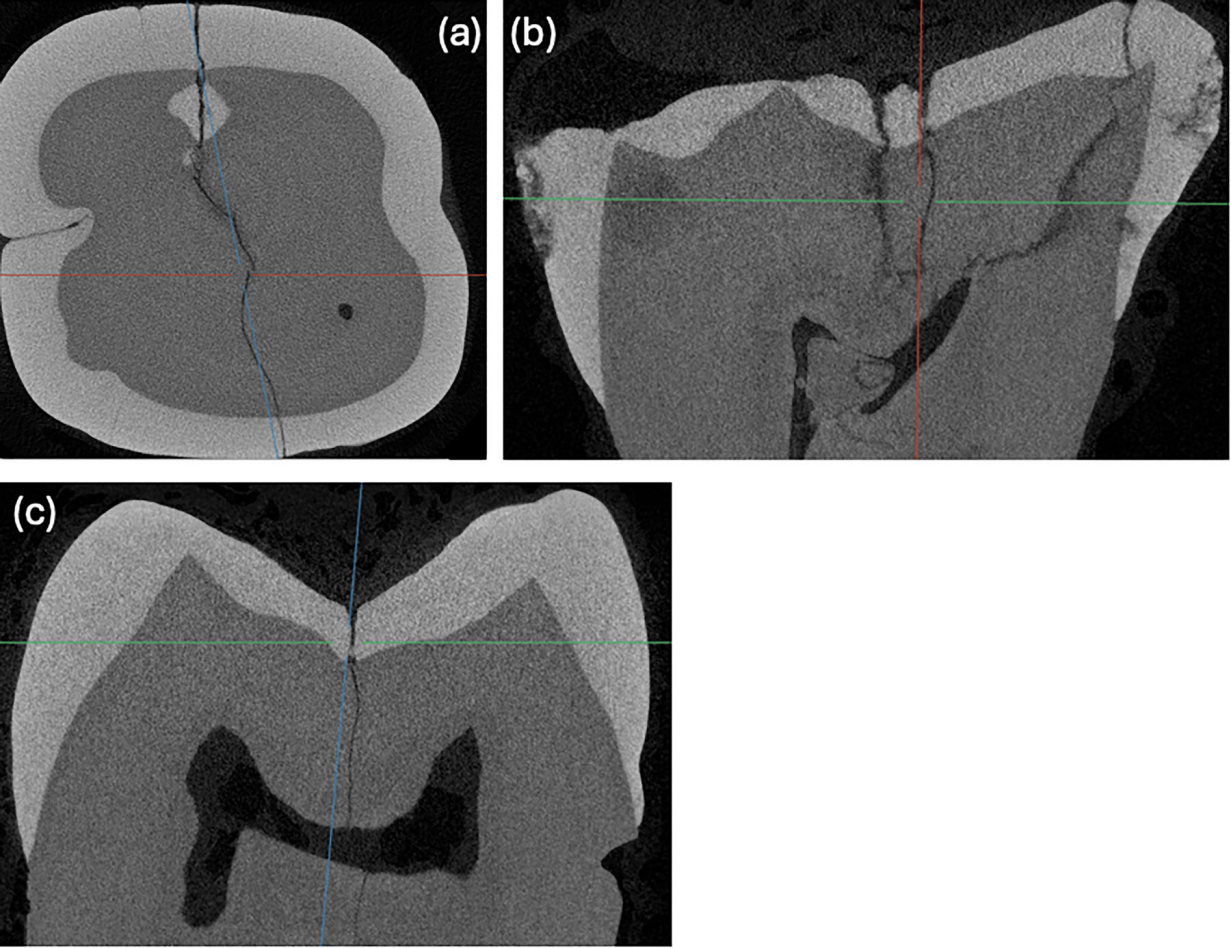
Micro‐CT scans of a sample tooth: (a) axial view, (b) sagittal view, and (c) coronal view.
